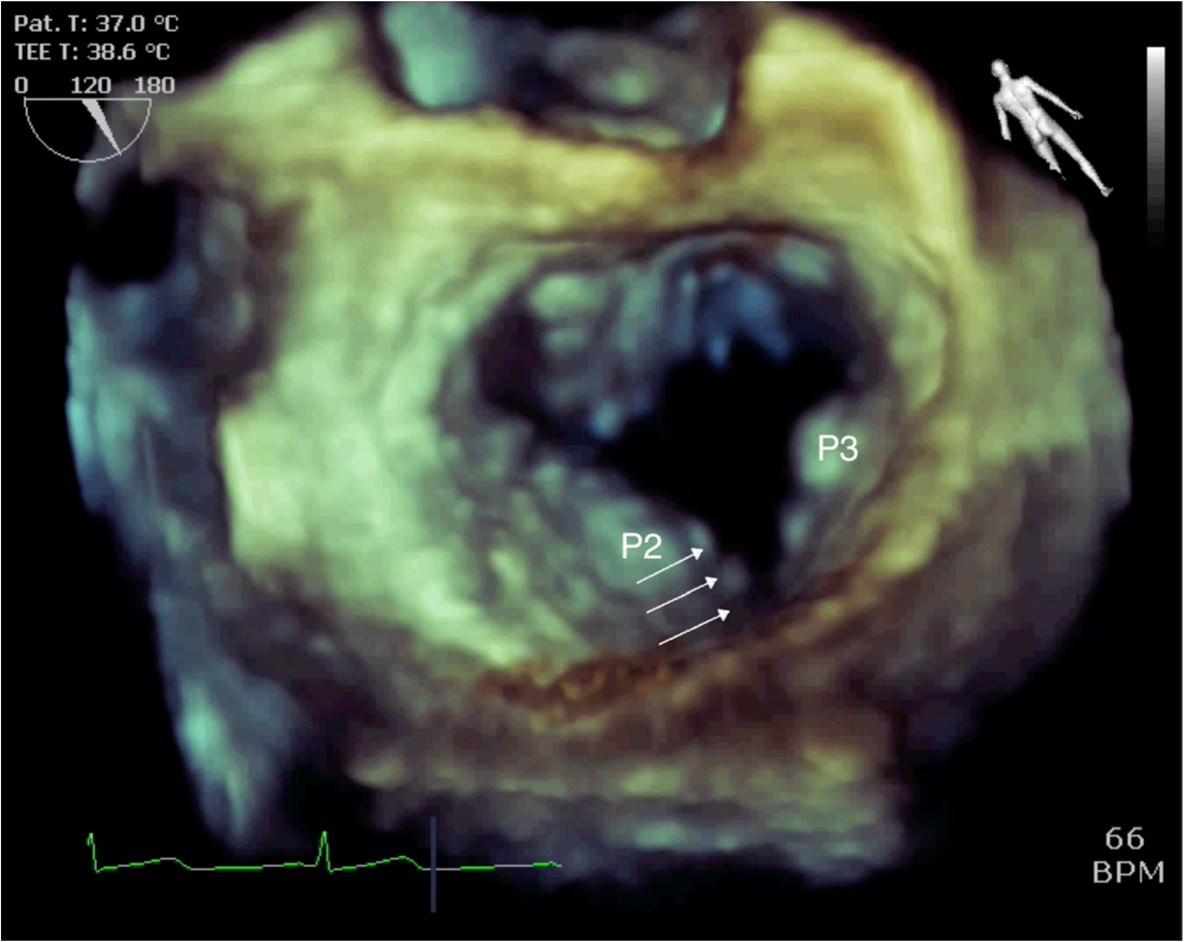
Three-dimensional echocardiography at the en face view at early diastole showing a profound indentation (cleft) between P2 and P3 (white arrow)
